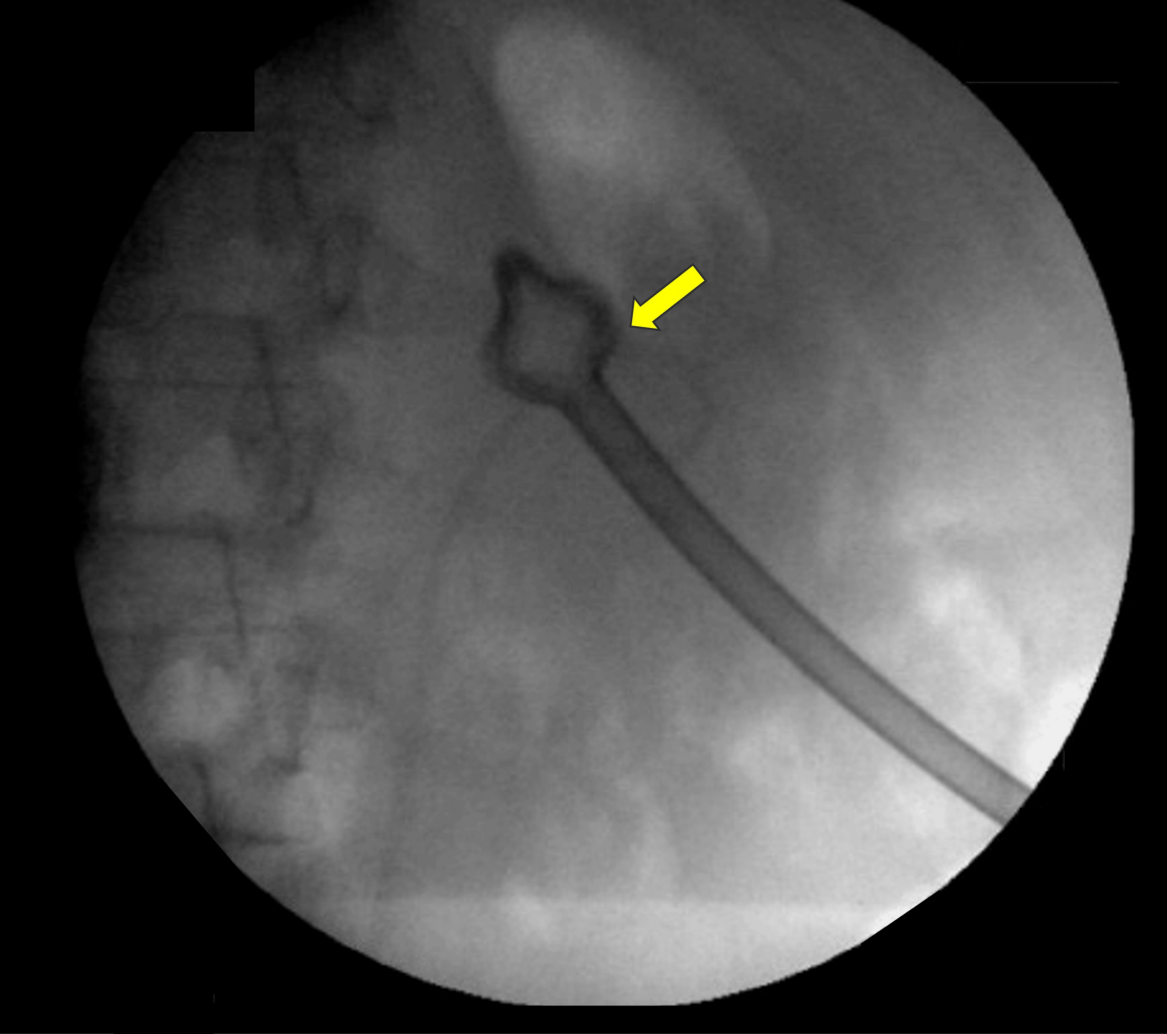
Intraoperative X-ray at the end of the PNL procedure showing placement of the Malecot catheter and the JJ stent (arrow). PNL, percutaneous nephrolithotomy; JJ, double-J
